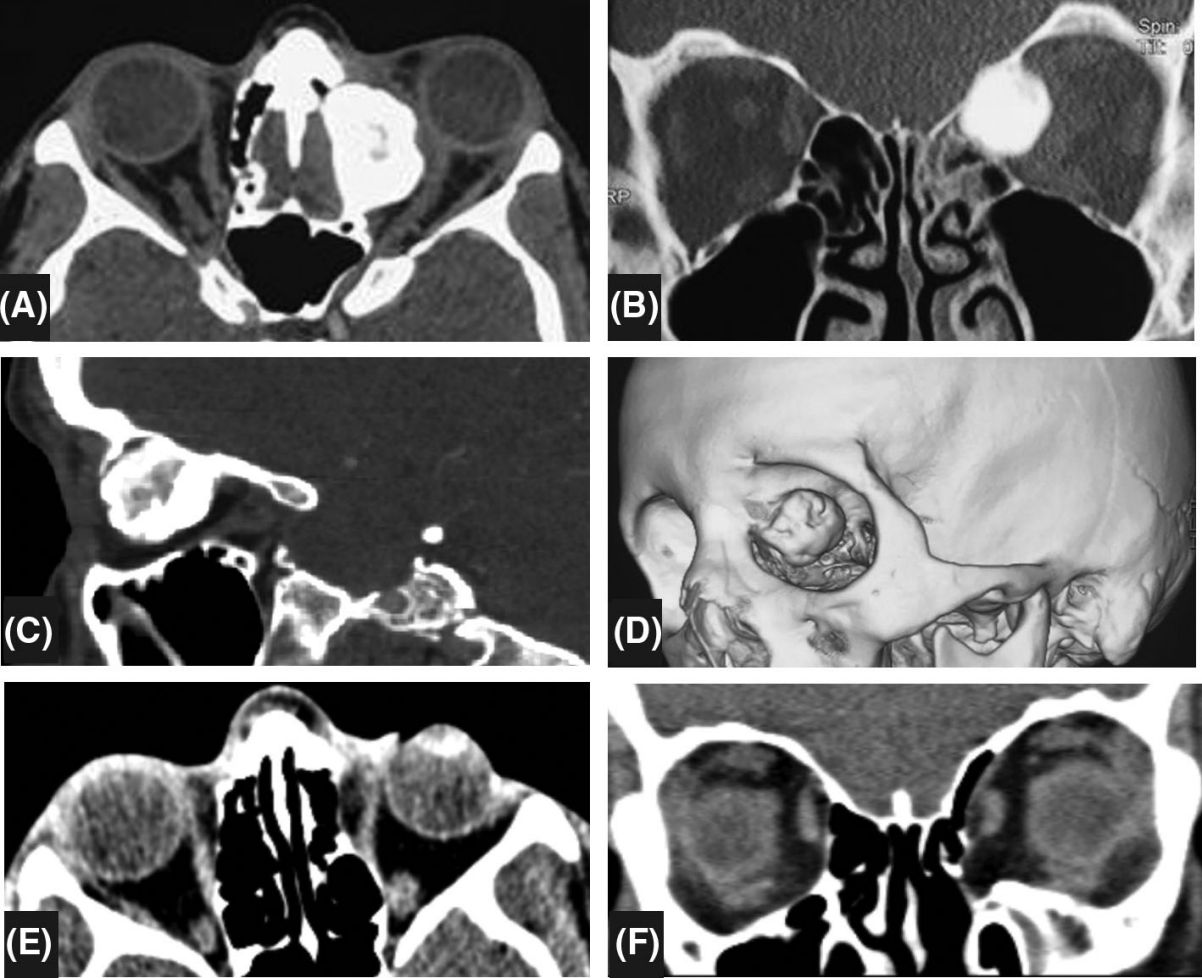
Orbital CT scan of the patient. At presentation, a superonasal giant osteoma is seen at the junction of the left frontal bone and the ethmoidal sinus, extending to the extraconal space of the left orbit. Frontal sinuses are not pneumatized and left ethmoidal sinus is opaque and partially obliterated. Compressive effect of the mass is seen on the optic nerve and orbital soft tissues. A, axial view; B, coronal view; C, sagittal view; D, three dimensional oblique view. At final follow up, the orbital walls are intact excepting the lamina papyracea and the soft tissues have returned to normal position E and F
